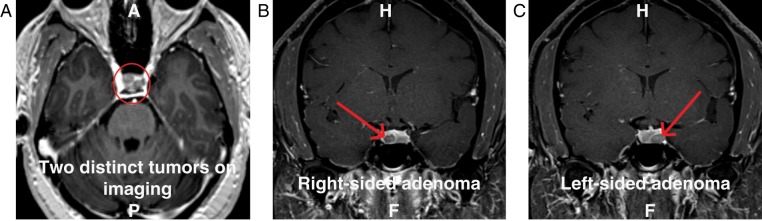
MRI demonstrating two distinct pituitary adenomas. (A) Axial MRI with contrast. (B) Coronal MRI with contrast. The arrow indicates the right-sided tumor. (C) Coronal MRI with contrast. The arrow indicates the left-sided tumor.
